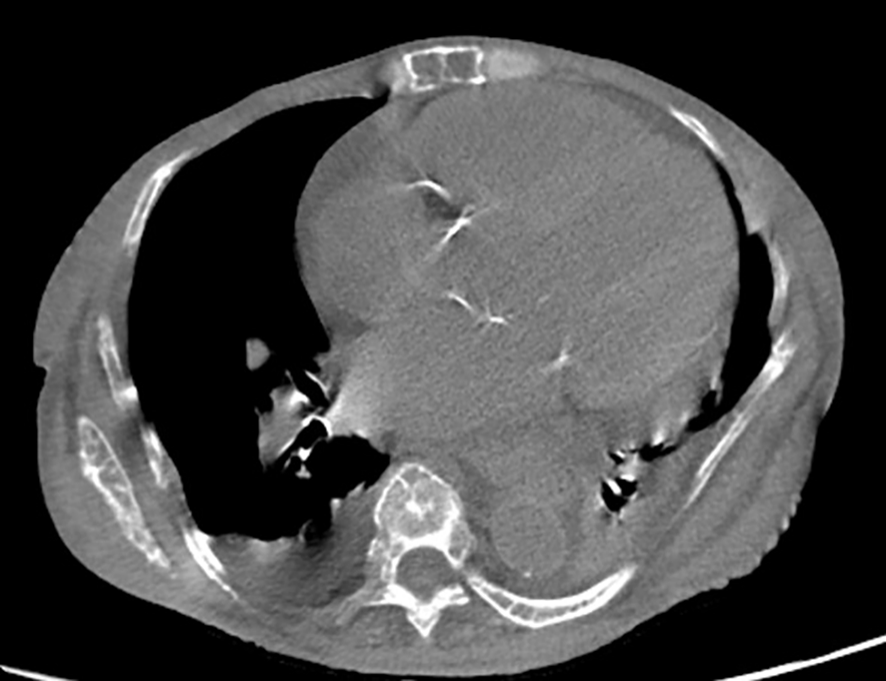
Non-contrast axial computed tomography in a soft tissue window at the level of the cardiac base reveals calcifications in the region of the heart valves. Incidentally, bilateral pleural effusion and passive atelectasis are observed.
